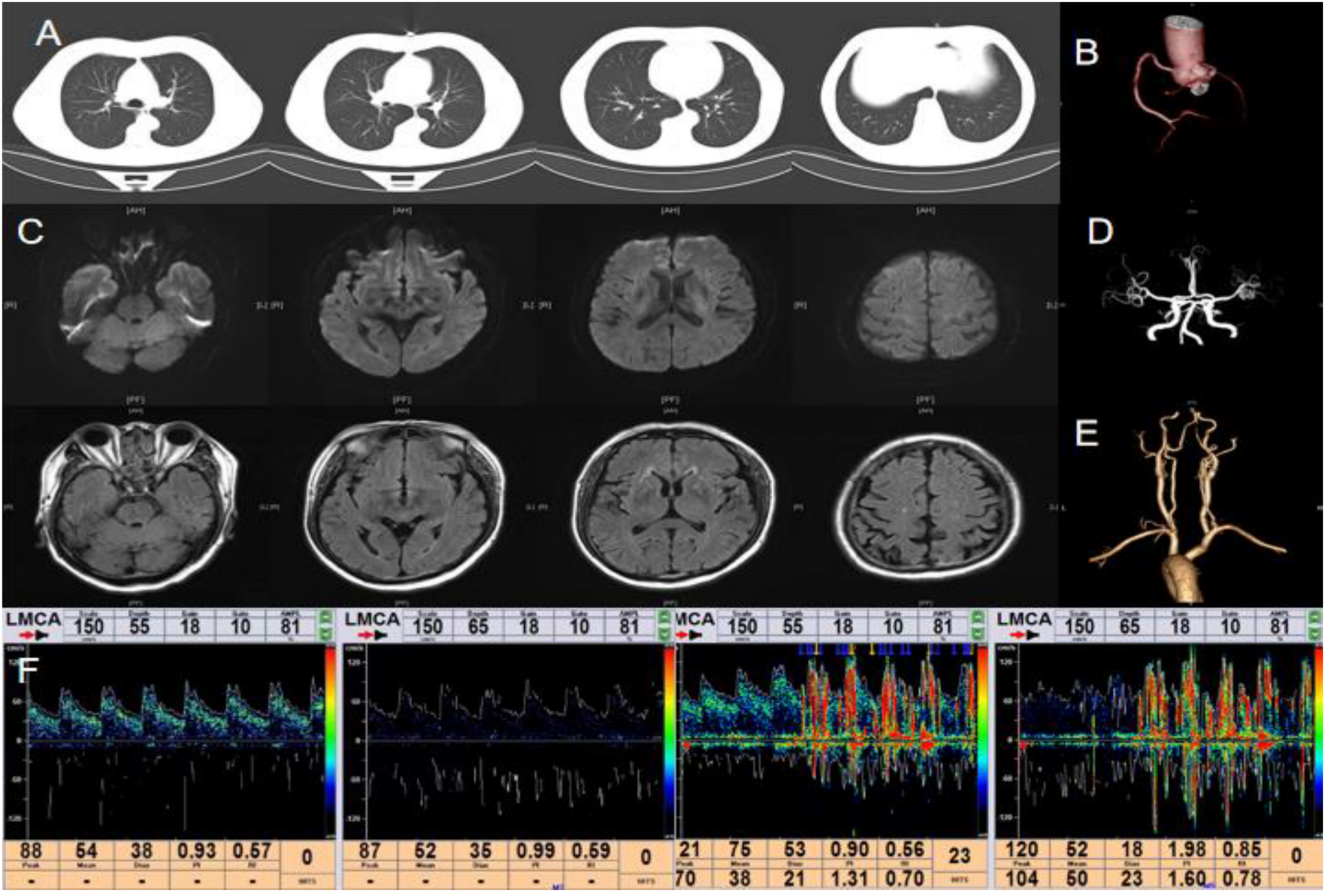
Main examination findings for case 3: (A) chest CT; (B) coronary CTA; (C) cranial MRI; (D) cranial MRA; (E) carotid CTA; (F) transcranial Doppler ultrasound bubble test.
